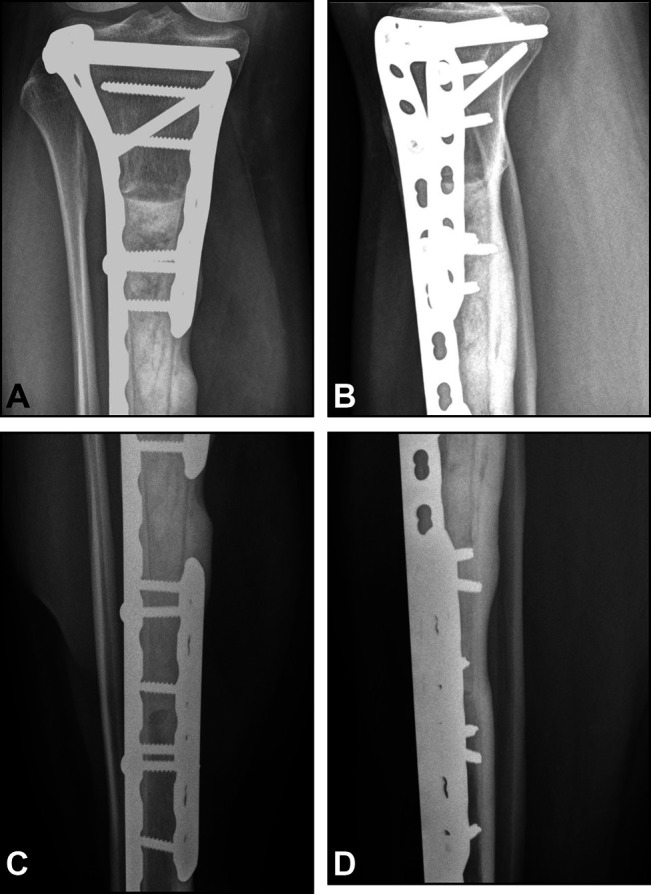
Radiograph showing AP and lateral views. A, Case 2: AP radiograph of proximal tibia and previous allograft fracture 34 months postoperatively demonstrating union of host-allograft junction and allograft fracture. B, Case 2: lateral radiograph of proximal tibia and previous allograft fracture 34 months postoperatively demonstrating union of host-allograft junction and allograft fracture. C, Case 2: AP radiograph of distal tibia and previous allograft fracture 34 months postoperatively demonstrating union of host-allograft junction and allograft fracture. D, Case 2: lateral radiograph of distal tibia and previous allograft fracture 34 months postoperatively demonstrating union of host-allograft junction and allograft fracture.
